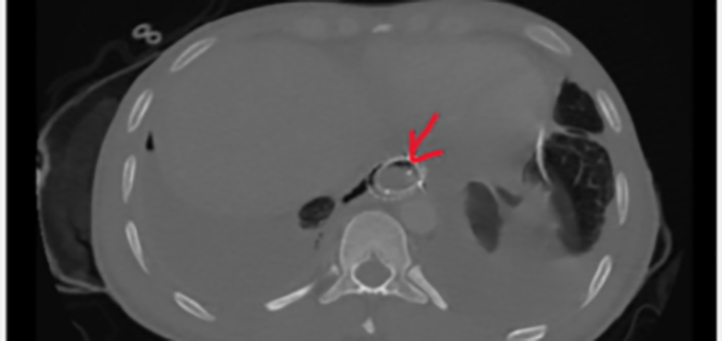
CT-lung window showing two overlapping CSES marked with an arrow.
